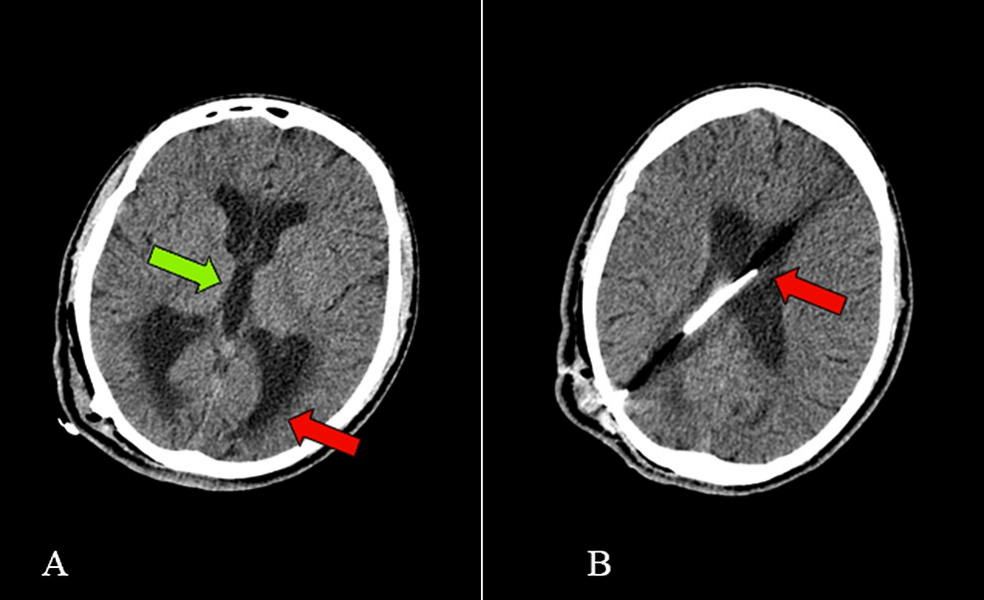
Hydrocephalus and proximal catheter (A) Axial section of a CT scan showing dilated lateral ventricles (red arrow) and dilated third ventricle (green arrow). (B) Axial section of a CT scan showing the position of proximal catheter (red arrow).
